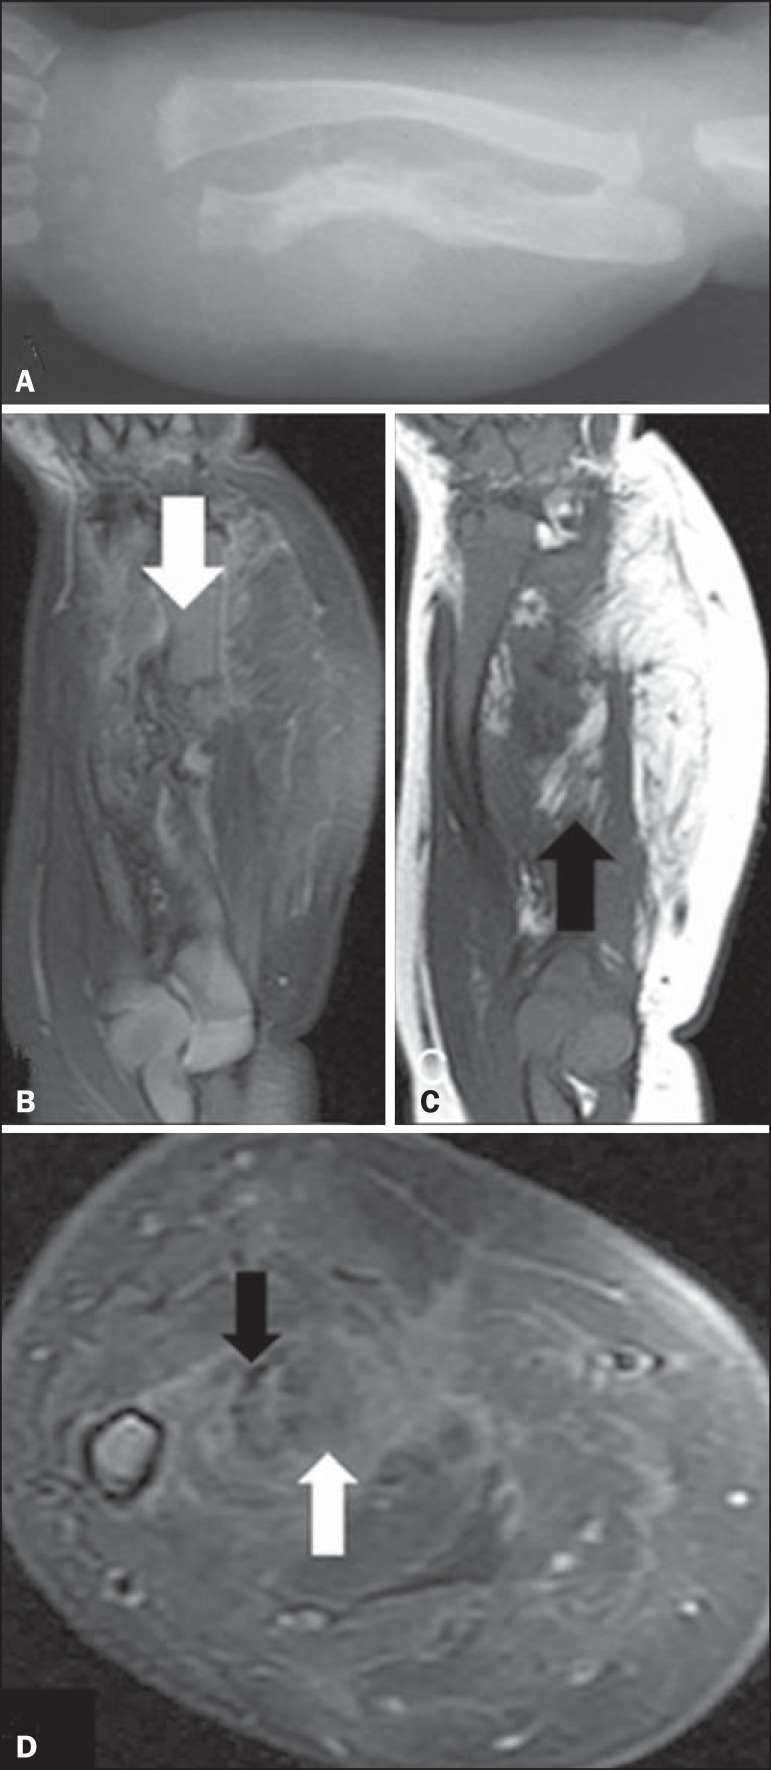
A: Forearm X-ray showing fracture associated with ulna
